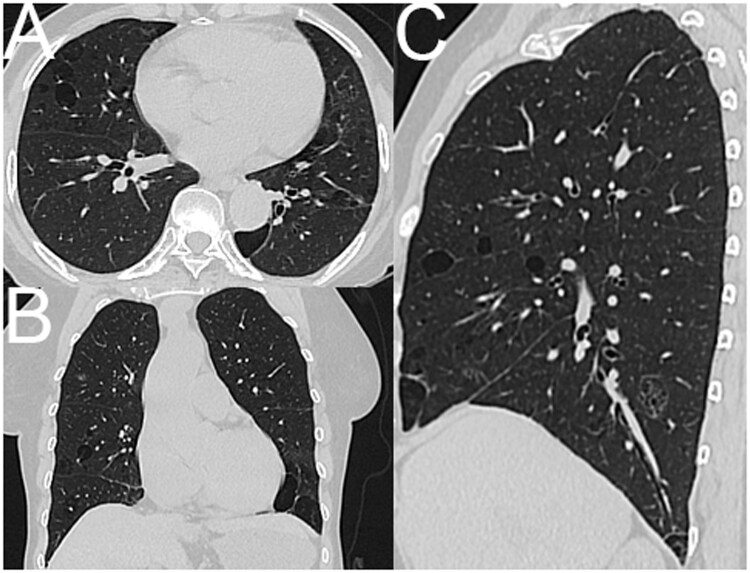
AMP in a 67-year-old nonsmoking woman with systemic lupus erythematosus. CT images (A-C) show multiple thin-walled cysts of variable size in the right middle lobe and both lower lobes, predominantly subpleural. Abbreviation: AMP = alveolar macrophage pneumonia.
